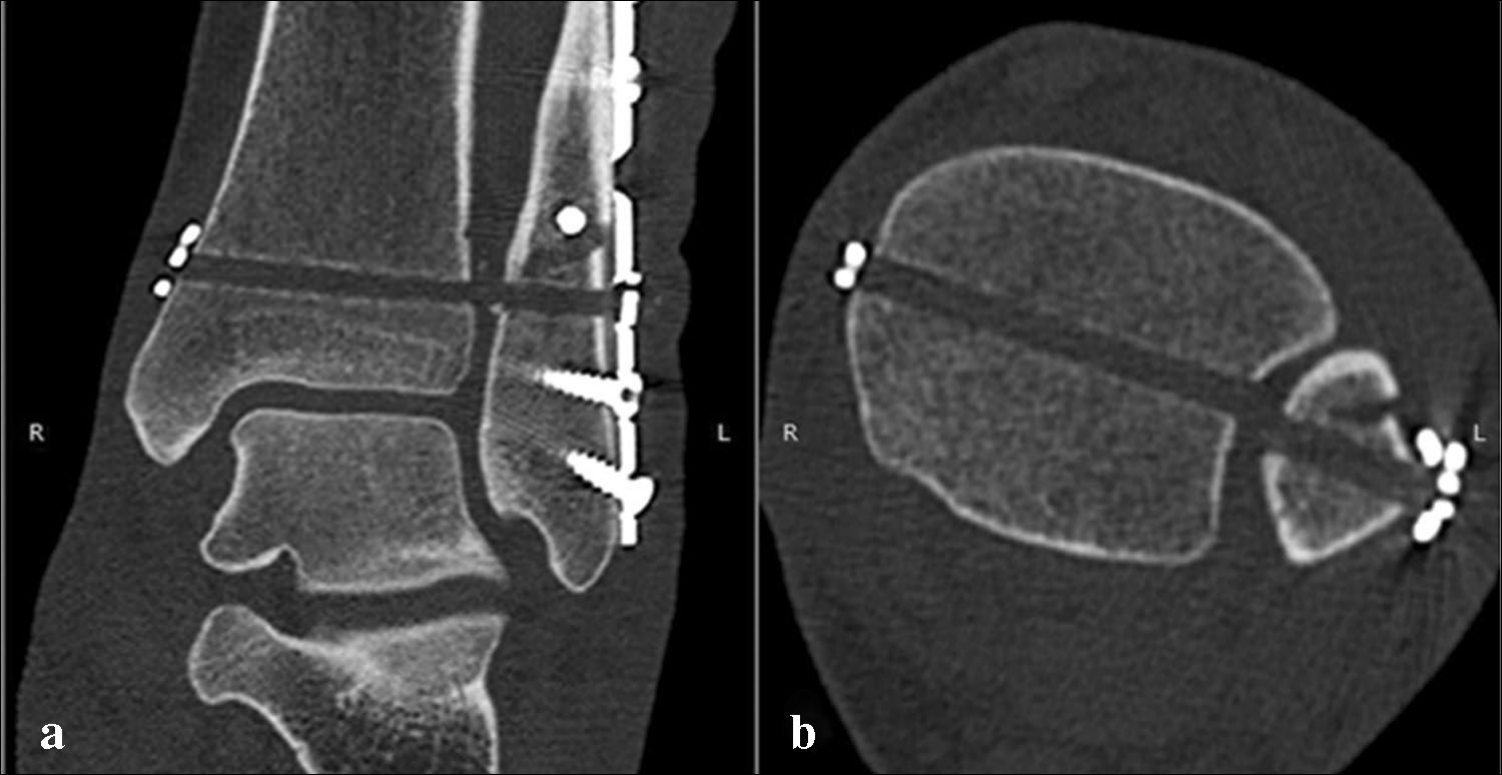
Computed tomography in axial (a) and coronal (b) reconstruction of a left ankle fracture classified as AO 44B3.1 after open reduction and internal fixation of the lateral malleolus (lag screw and neutralization plate) and dynamic stabilization of the distal tibiofibular joint
